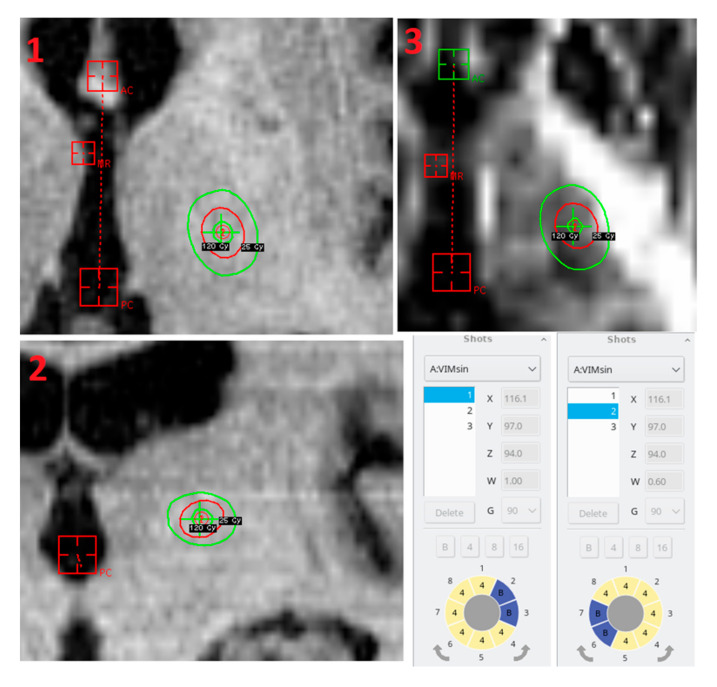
Shot in the shot radiosurgery technique for the left nucleus ventralis intermedius (VIM) thalamotomy, 1—axial plane, 2—coronal plane, 3—MRI tractography (ep2d_diff) axial plane.
